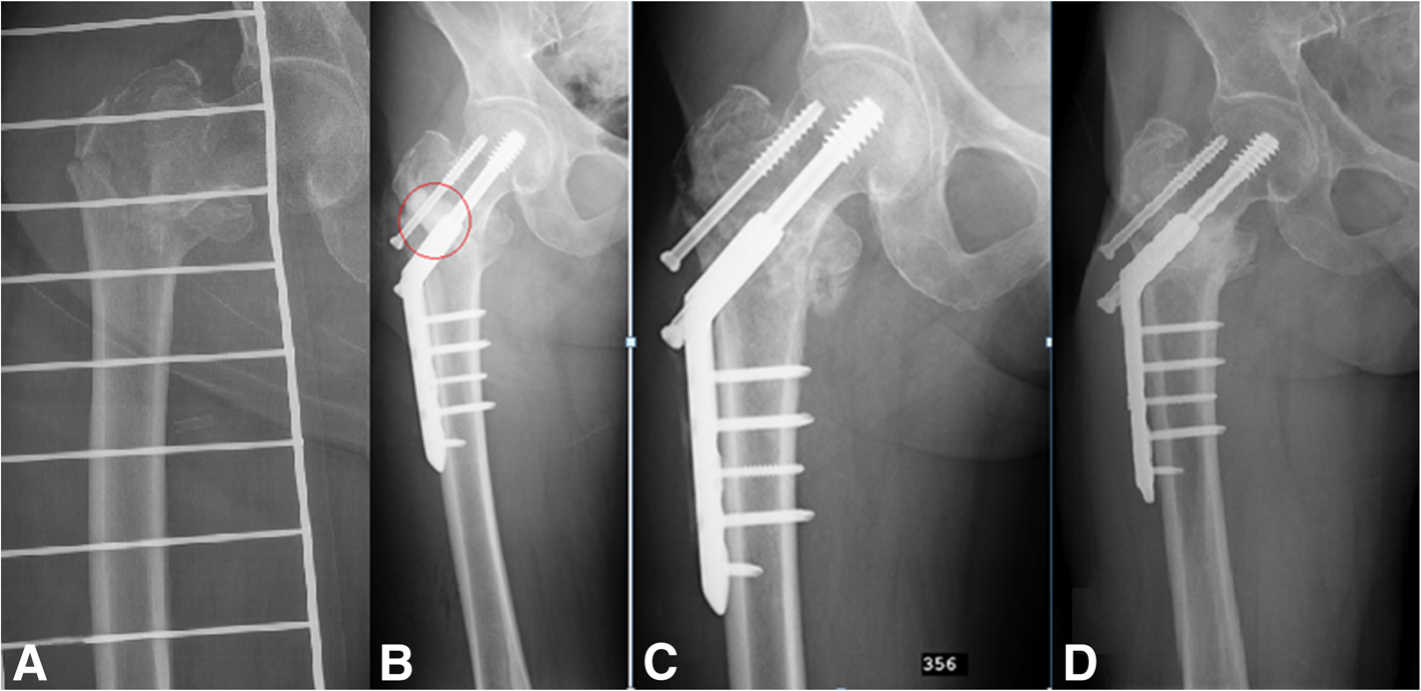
DHS patient‘s proximal part of the femur healing. Preoperative image shows the trochanteric fracture (a). In the postoperative image (b) CERAMENT™|G is seen (circled in red). In the X-ray image after 3 months (c), the bone graft substitute is difficult to differentiate from bone. Image after 1 year (d) shows complete healing. This patient needed DHS removal because of local discomfort
